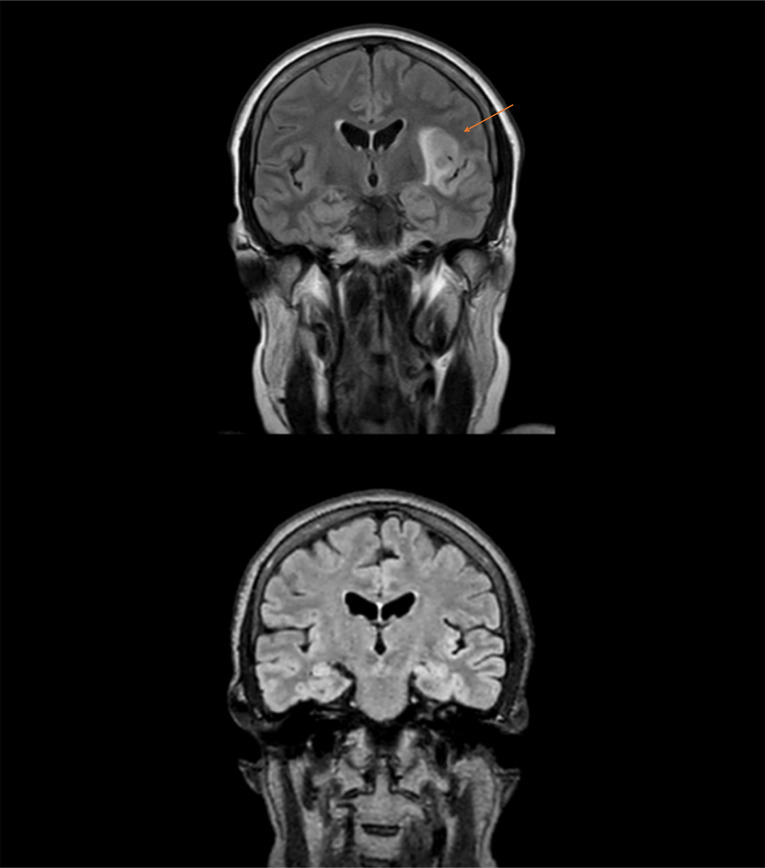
Brain MRI (1.5 Tesla, slice thickness 5 mm) with fluid-attenuated inversion recovery (FLAIR) sequence demonstrating a left-hemispheric peri-insular lesion (red arrow) and complete regression of the lesion at 3-month follow-up.
